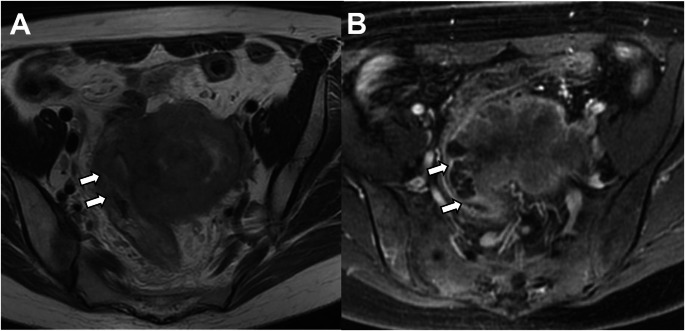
Stage IVA grade 3 endometrioid adenocarcinoma in a 77-year-old woman. A Axial T2-weighted fast spin-echo (FSE) image (TR/TE: 4500/100 ms; slice thickness: 3 mm) and B axial contrast-enhanced 3D T1-weighted fat-suppressed LAVA sequence (TR/TE: 4.2/2.1 ms; slice thickness: 2 mm; post-gadolinium, delayed phase at 4 min) show a large endometrial mass with myometrial invasion, extending through the myometrium and serosal surface directly into the rectal mucosa (arrows). Best seen on delayed contrast-enhanced image
